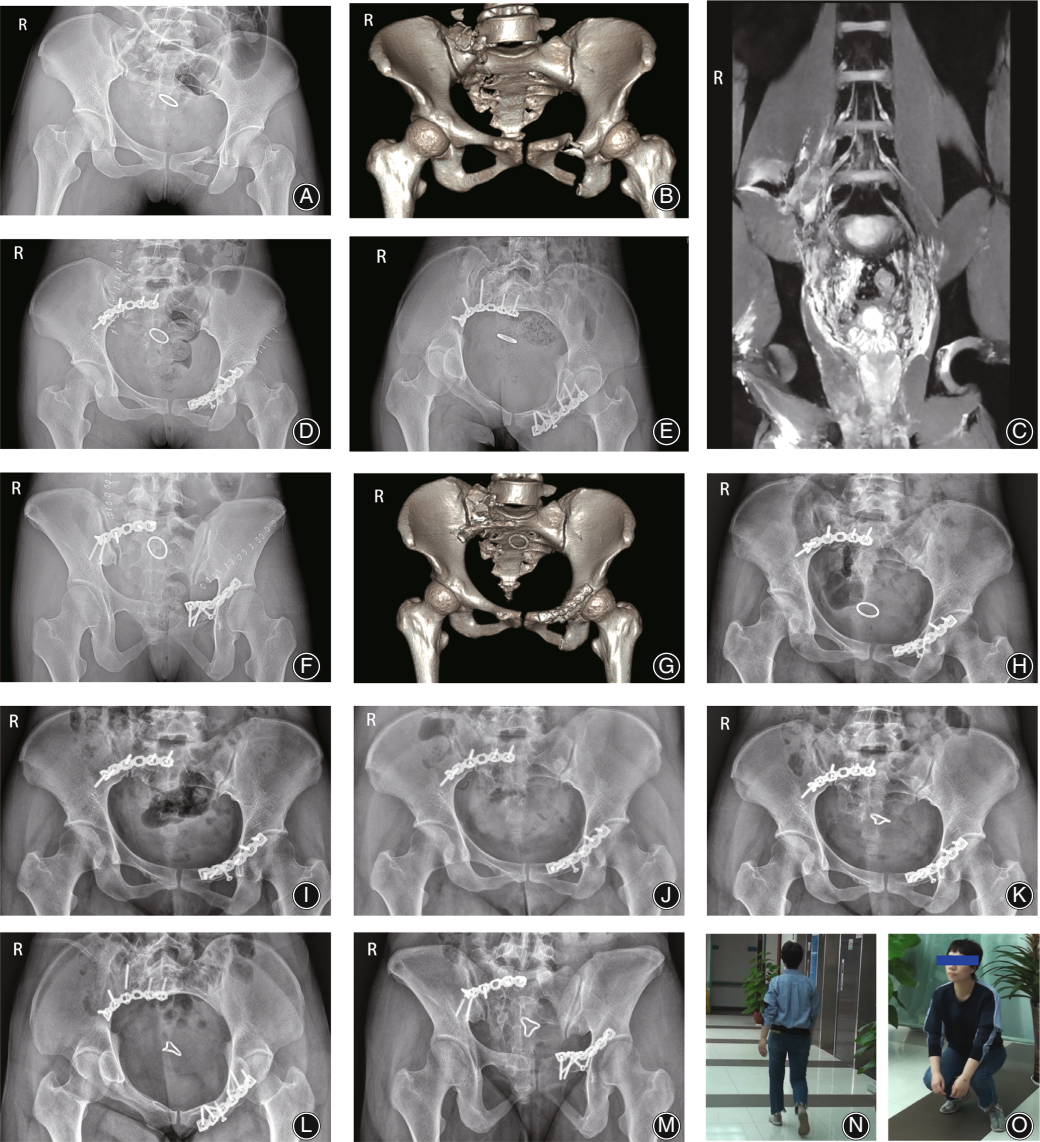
(A) Preoperative AP view; (B) Preoperative 3‐D reconstruction of CT data; (C) Preoperative MRI; (D–F) Postoperative AP, inlet and outlet views; (G) Postoperative 3‐D reconstruction of CT data; (H) Postoperative AP view at 3‐month follow‐up; (I) Postoperative AP view at 2‐year follow‐up; (J) Postoperative AP view at 4‐year follow‐up; (K–M) Postoperative AP, inlet, and outlet views at 8‐year follow‐up; (N–O) The patient could walk, run, and jump normally without complaints of sacroiliac pain at 8‐year follow‐up
